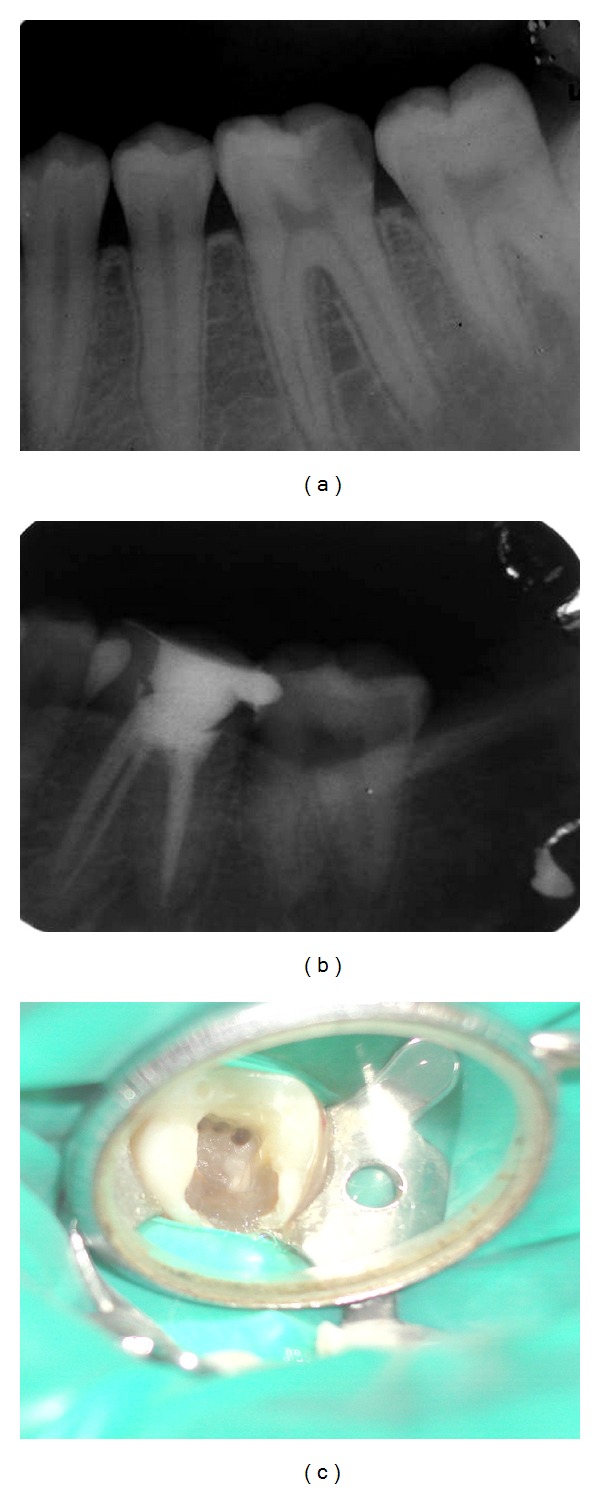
(a) Preoperative radiograph. (b) Obturation. (c) Clinical image.
